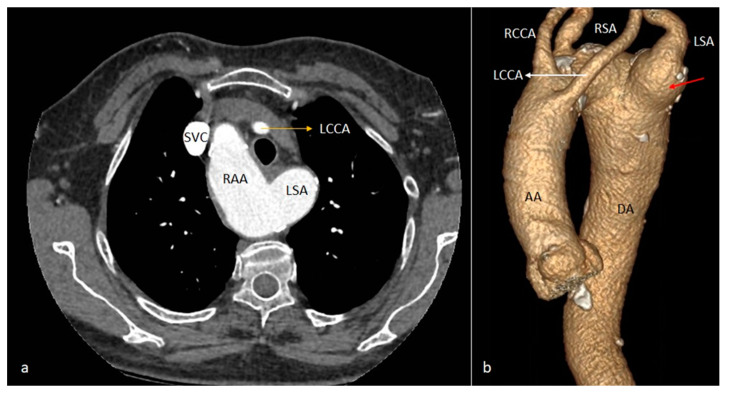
Axial CT (a) and VR (b) images showing a type II right aortic arch (RAA) and the aberrant origin of the left subclavian artery (LSA). Kommerell’s diverticulum shows as a focal ectasia at the base of the LSA origin (red arrow). SVC—superior vena cava. RAA—right aortic arch. LCCA—left common carotid artery. LSA—left subclavian artery. RCCA—right common carotid artery. RSA—right subclavian artery. AA—ascending aorta. DA—descending aorta.
